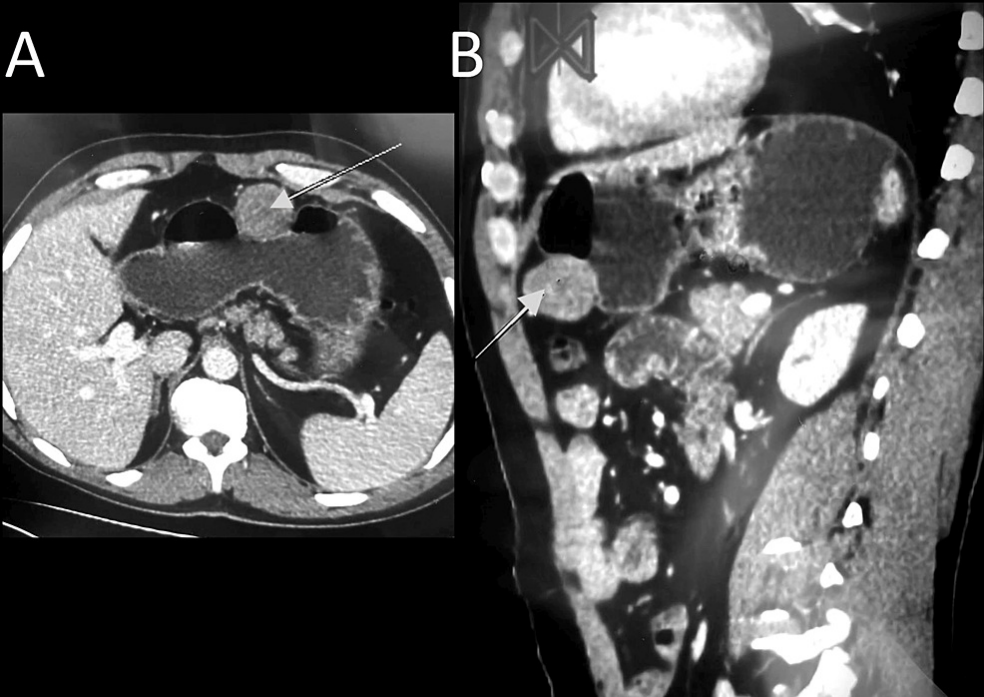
Abdominal CT scan transverse plane (A) and sagittal plane (B) showing homogenous and enhanced vascular mass (arrows) that arose from a splanchnic branch, it was adjacent to the anastomosis performed before, suggesting a pseudoaneurysm of the GDA.
